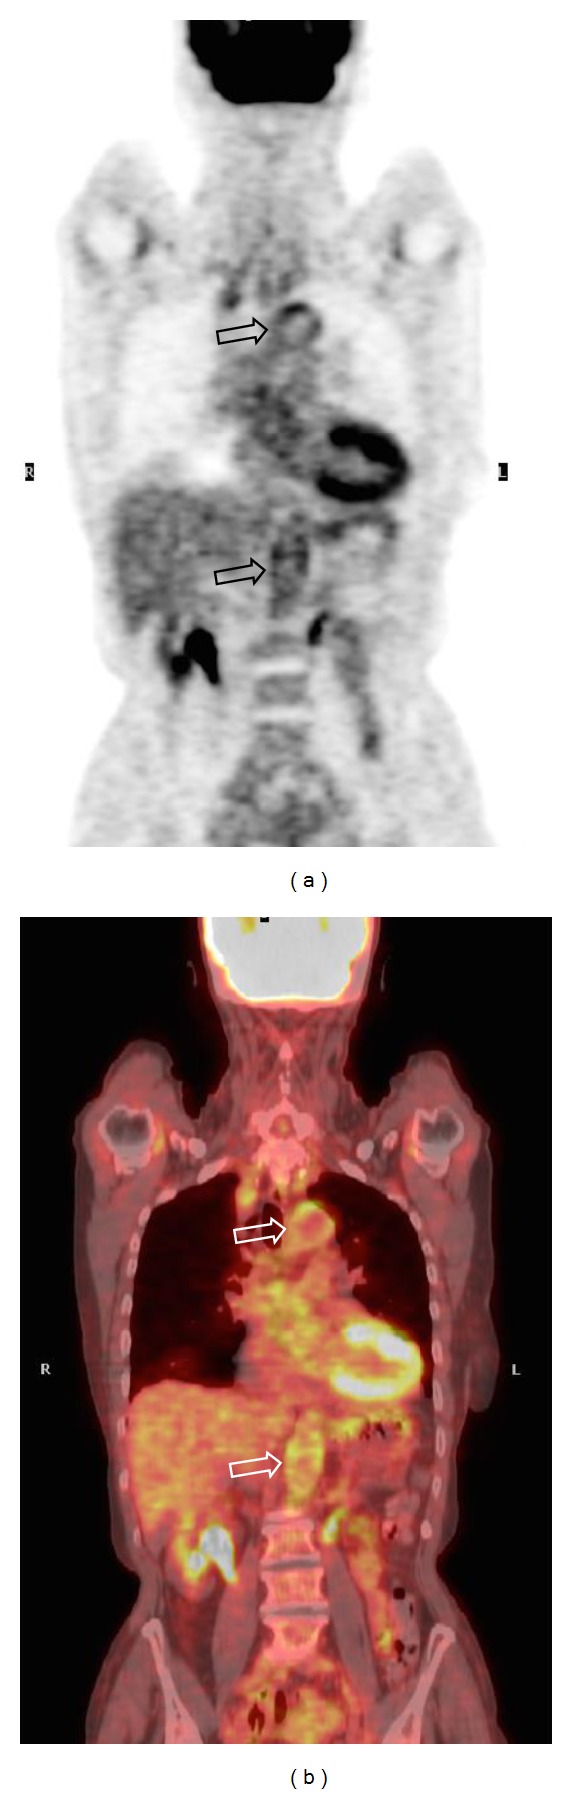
77-year-old female patient with clinical and PET-CT findings of giant cell arteritis. Coronal PET (a) and PET-CT (b) scans demonstrate the inflammatory involvement of aortic arch and abdominal aorta (void arrows), which is clearly appreciable by means of an immediate qualitative assessment of the images.
